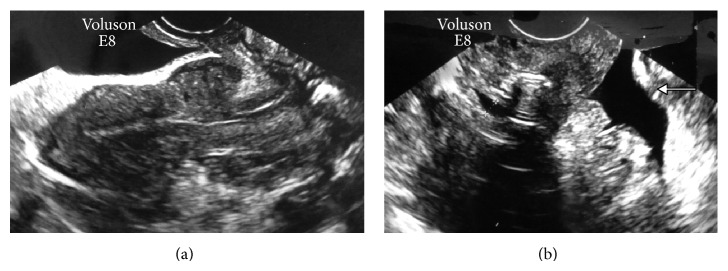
Ten months after the cesarean section. (a) Transvaginal ultrasonography shows a normal-sized uterus, but a clot-like mass with a low-to-iso-echoic inhomogeneous content, measuring 20 × 15 mm, is shown at a location corresponding to the site of a uterine incision in the vesicouterine pouch. (b) Saline infusion sonohysterography was performed using a physiological saline solution. During the saline infusion the uterine lumen did not expand and a fistula, measuring 4 mm in diameter, was observed (+- - -+). The images show that the physiological saline solution flowed out and spread from the uterine cavity into the peritoneal cavity through the vesicouterine pouch. In addition, the findings show that when the infusion continued, the saline solution accumulated in the Pouch of Douglas (arrow).
